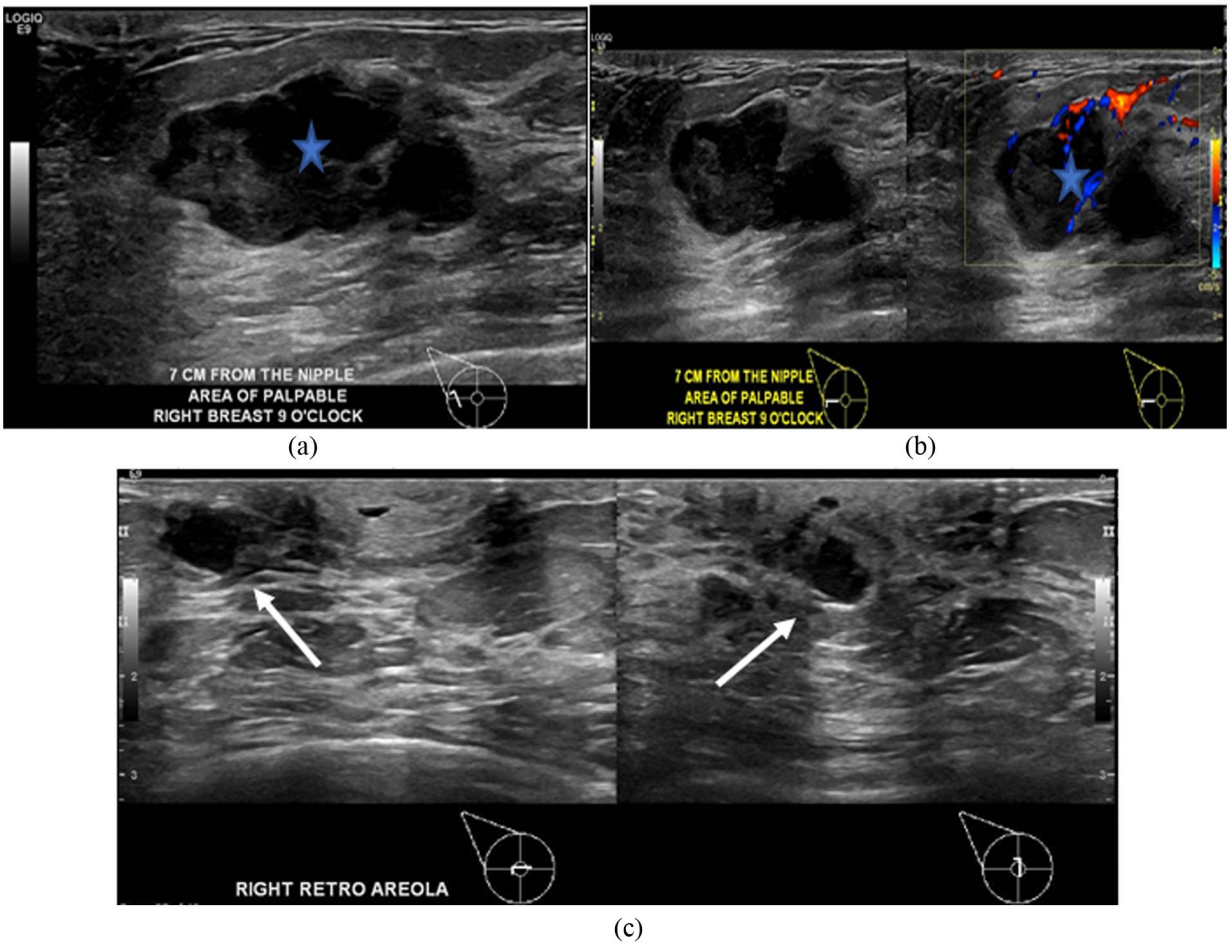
Right breast ultrasound showed two hypoechoic oval masses with lobulated margins and an intra-cystic component extending to the nipple with possible intraductal position of the masses. (a) Showed a hypoechoic mass (asterisk) with lobulated margins at 9:00, 7 cm from nipple. (b) Showed mass with significant intralesional vascularity (asterisk). (c) Showing the extension of the mass toward the base of the nipple with possible dilated ducts (arrow).
